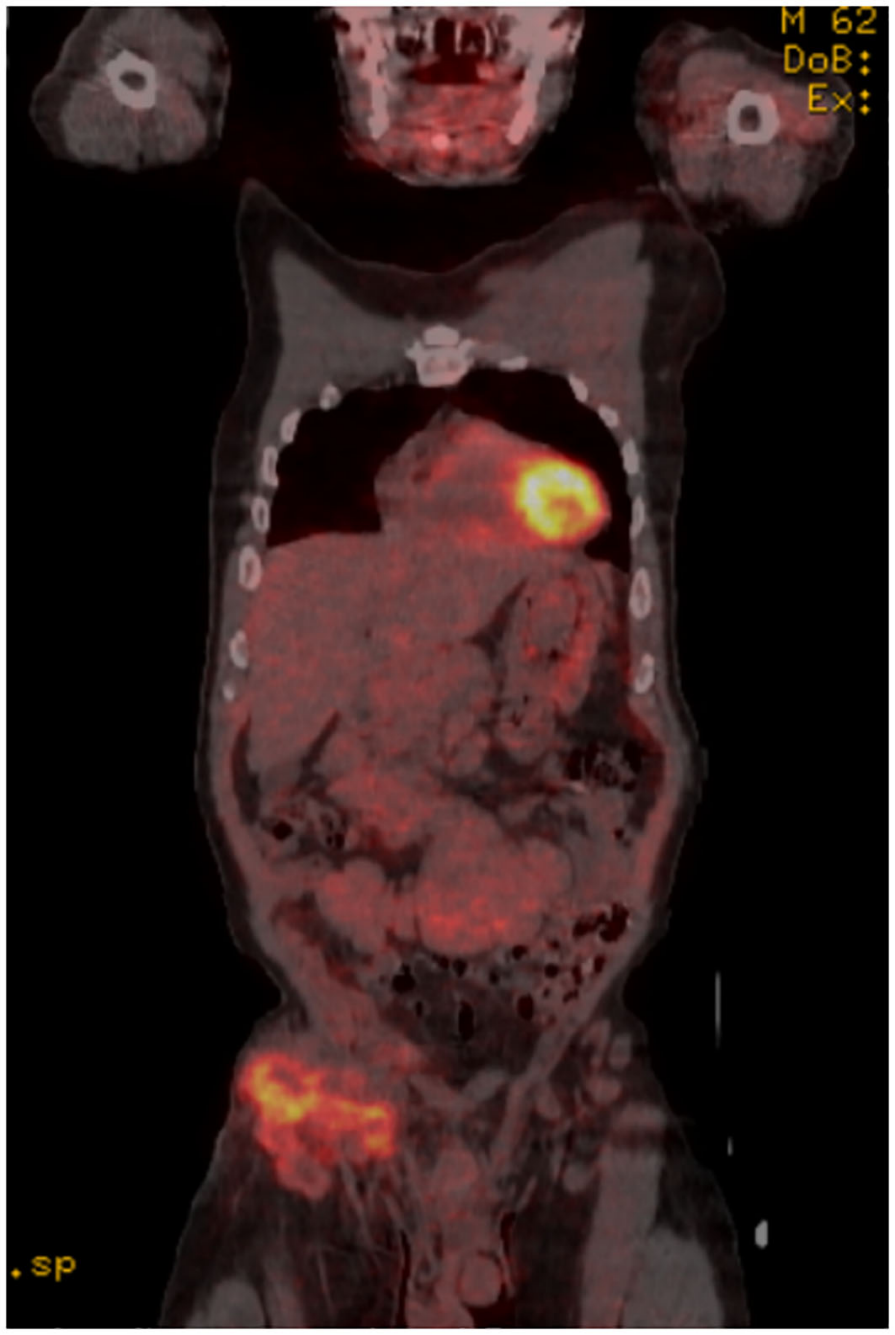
Coronal PET/CT scan showing high fluorodeoxyglucose (FDG) uptake in the R inguinal node.
